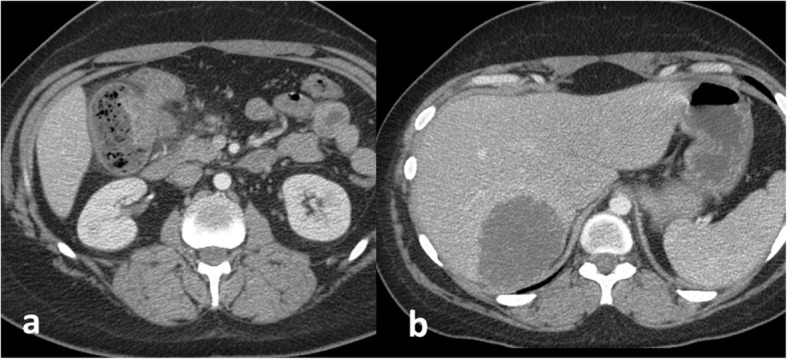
29-year-old female with Lynch Syndrome presents with abdominal pain and bloody diarrhea. a. Axial contrast enhanced CT image of the abdomen demonstrates a partial bowel obstruction secondary to a primary colonic neoplasm at the hepatic flexure with surrounding inflammatory changes suggestive of colitis. b. Large right hepatic mass measuring up to 8 cm consistent with metastatic disease
